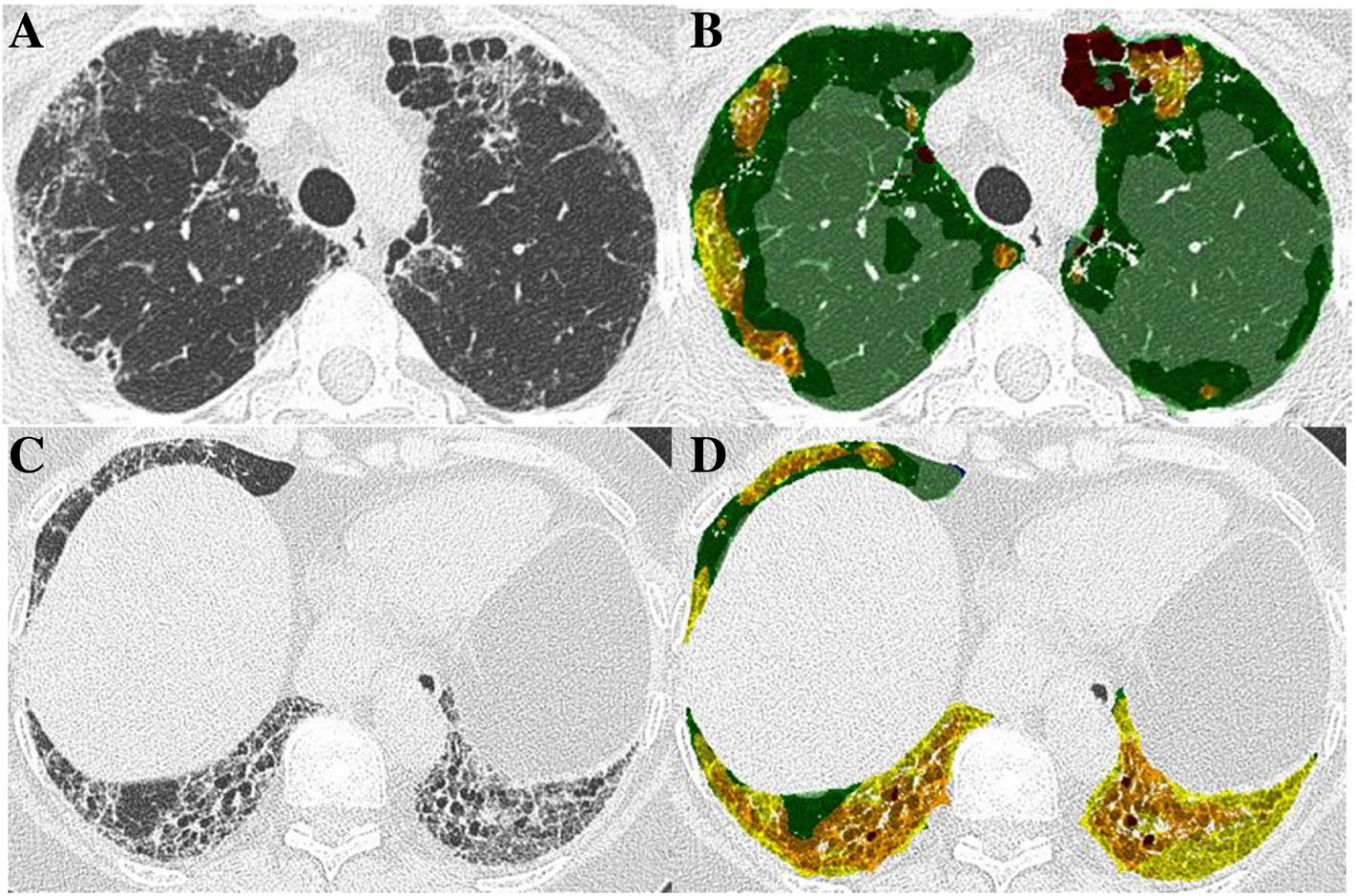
Axial CT image in a 46-year-old female never smoker (a), diagnosed with chronic hypersensitivity pneumonitis following surgical lung biopsy. An upper lobe predominant fibrosing lung disease associated with honeycomb cysts is demonstrated. On the CALIPER colour overlay image (b), ground glass opacities (yellow) are interspaced with reticular pattern (orange) in the lung periphery. In the anterior left upper lobe, there are honeycomb cysts visible (brown). Light and dark green areas represent normal lung parenchyma. Axial CT image in a 55-year-old female 2-pack-year ex-smoker (c), diagnosed with chronic hypersensitivity pneumonitis following surgical lung biopsy with more extensive fibrosis involving the lower lobes. CALIPER colour overlay images show minor misclassification of traction bronchiectasis as honeycomb cysts in the left lower lobe (d), but extensive ground glass opacities (yellow) and interspaced reticular pattern (orange)
